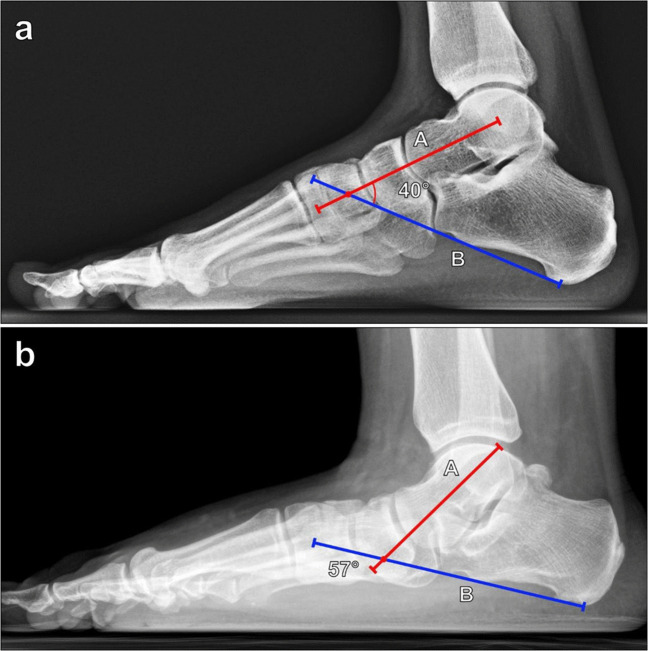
Measurement of the lateral talocalcaneal angle on lateral foot radiograph. a The angle is formed between a line (A, red line) along the axis of the talar neck and a line (B, blue line) along the inferior surface of the calcaneus. Normal values are between 25° and 40°. Values < 25° are seen in pes cavus, hindfoot varus, and congenital talipes equinovarus, while values > 40° are seen in pes planus and hindfoot valgus. b In a 34-year-old male patient with posterior tibialis tendon dysfunction and flatfoot deformity, the lateral talocalcaneal angle measures 57°, indicating pes planus and hindfoot valgus
